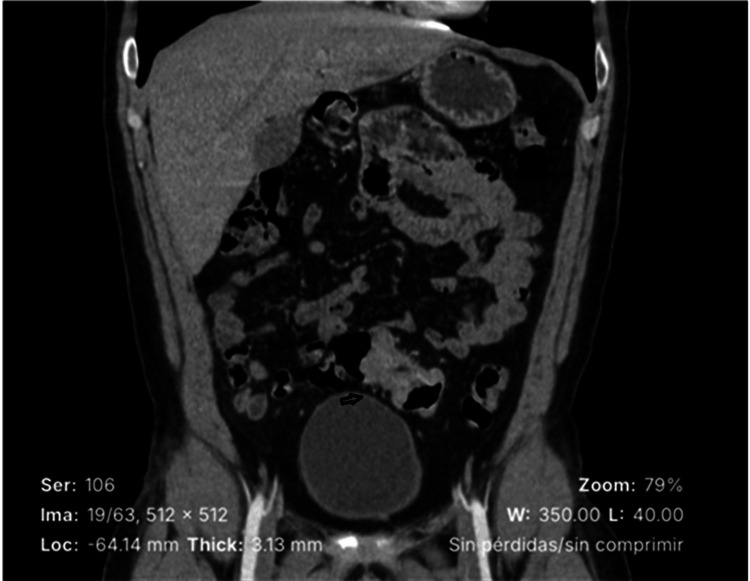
CT of the abdomen and pelvis with intravenous contrast CT demonstrates circumferential wall thickening of the sigmoid colon (arrow). CT: computed tomography
